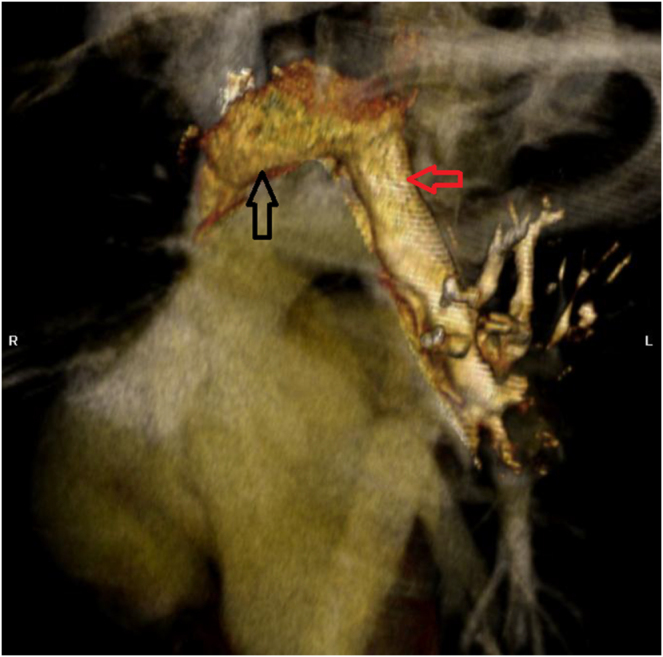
Preoperative CTA image showing the ascending vertical vein (The red Arrow) connecting into the Innominate vein (Black Arrow).
